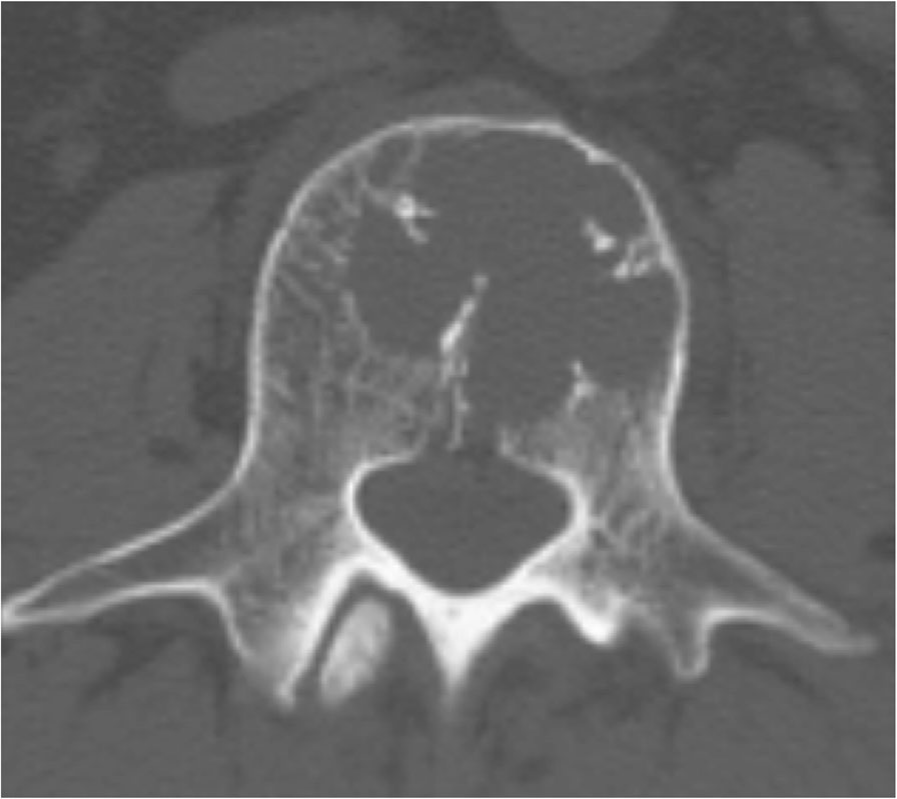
Preoperative computed tomography scan reveals osteolytic lesions in the anterior column of L3 vertebra
